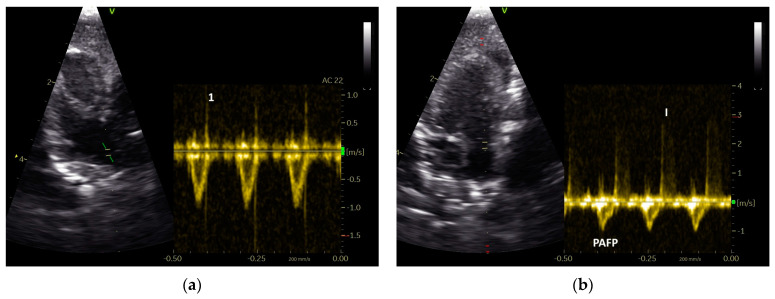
PW Doppler sonographic blood flow profiles of the pulmonary artery of a Congo grey parrot that developed right heart failure over the course of one year: (a) a symmetric blood flow profile of the pulmonary artery (PAFP), without signs of heart failure, and (b) the shape of the PAFP a year later, with a short acceleration phase seen in conjunction with the development of right heart failure displayed as right ventricular dilatation and an insufficiency of the pulmonary valve. I: insufficiency jet; 1: Doppler signal of the pulmonary valve, with a sample gate placed in the area of the pulmonary valve; AC: angle correction.
